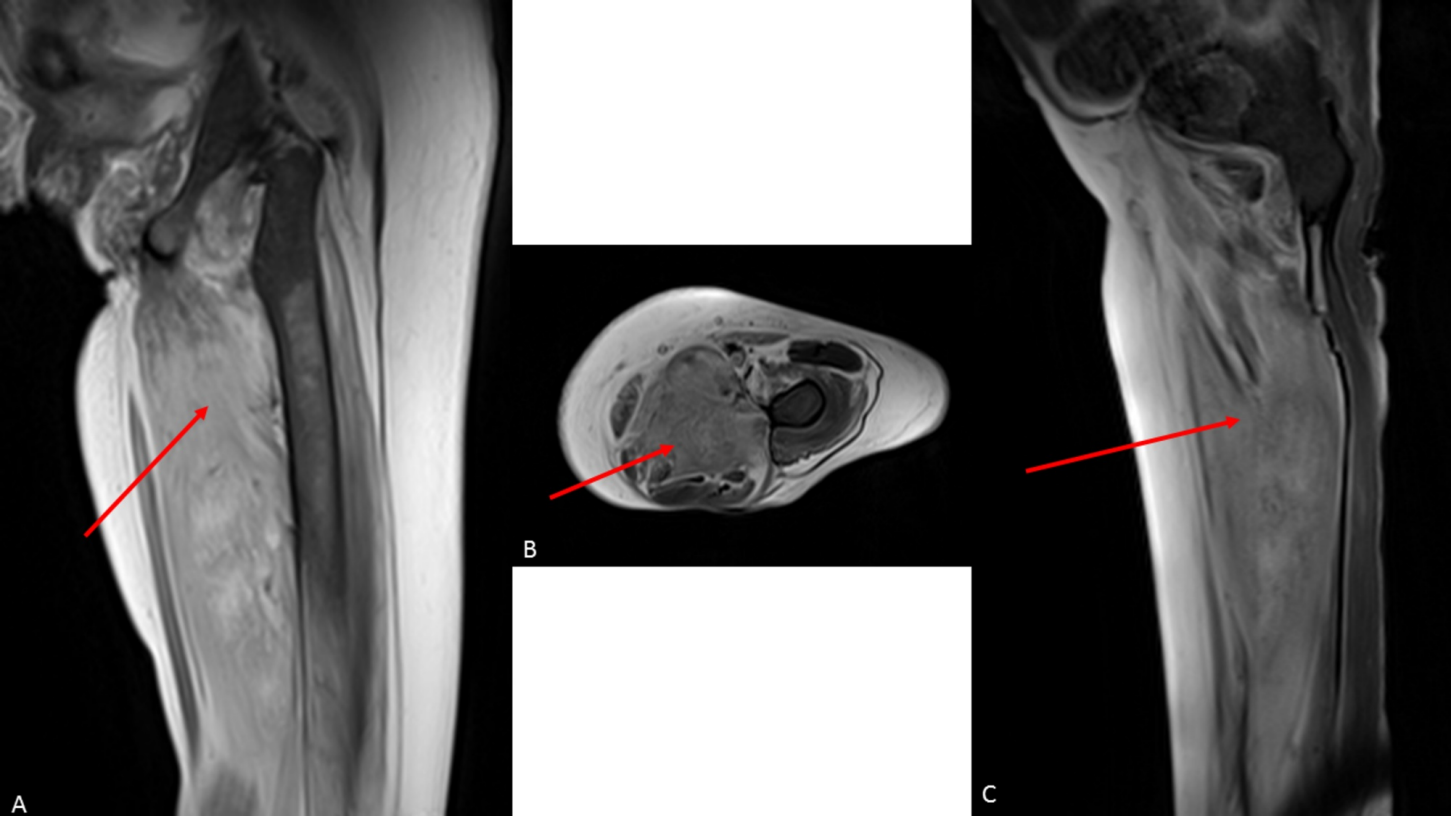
(A) MRI STIR coronal image, (B) MRI T2 fat saturated axial image, and (C) MRI T2 fat saturated sagittal image demonstrating diffuse subcutaneous edema and edema in thigh musculature that was most pronounced within the hamstring muscles (red arrows). MRI - magnetic resonance imaging, STIR - short-TI inversion recovery
